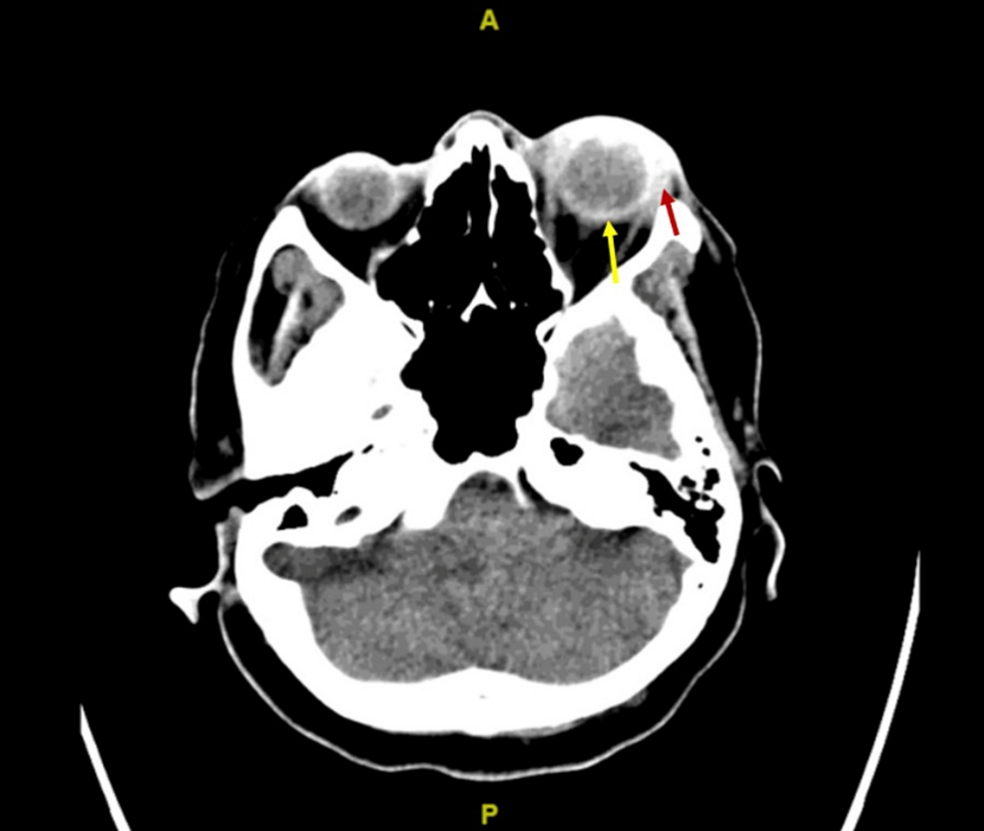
CT scan of the brain and orbit showing left globe proptosis, diffuse thickening of the sclera, choroidal enhancement (yellow arrow), and denser appearance of aqueous and vitreous humor of the left globe, as well as extensive left periorbital fat stranding (red arrow).
